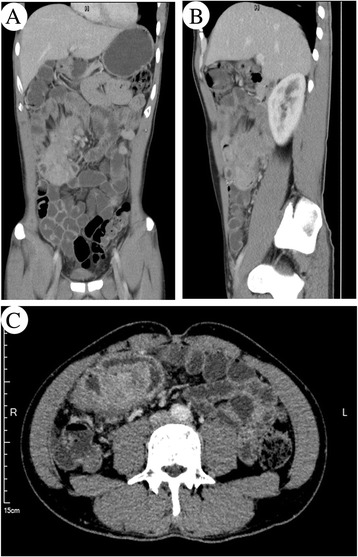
Computed tomography (CT) scan of an abdominal mass. CT showed a mass involved small intestines measured 8.5 × 7.4 × 4 cm in coronal view (a) sagittal view (b) and transverse section (c)
